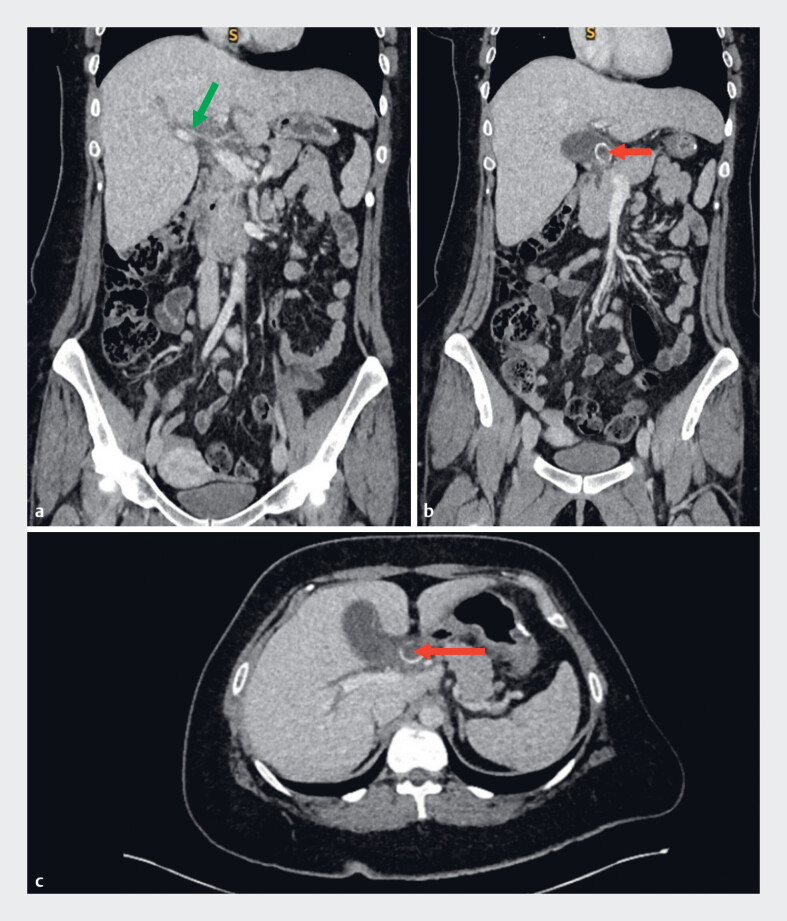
Abdominopelvic computed tomography scan before cholecystectomy showing:
